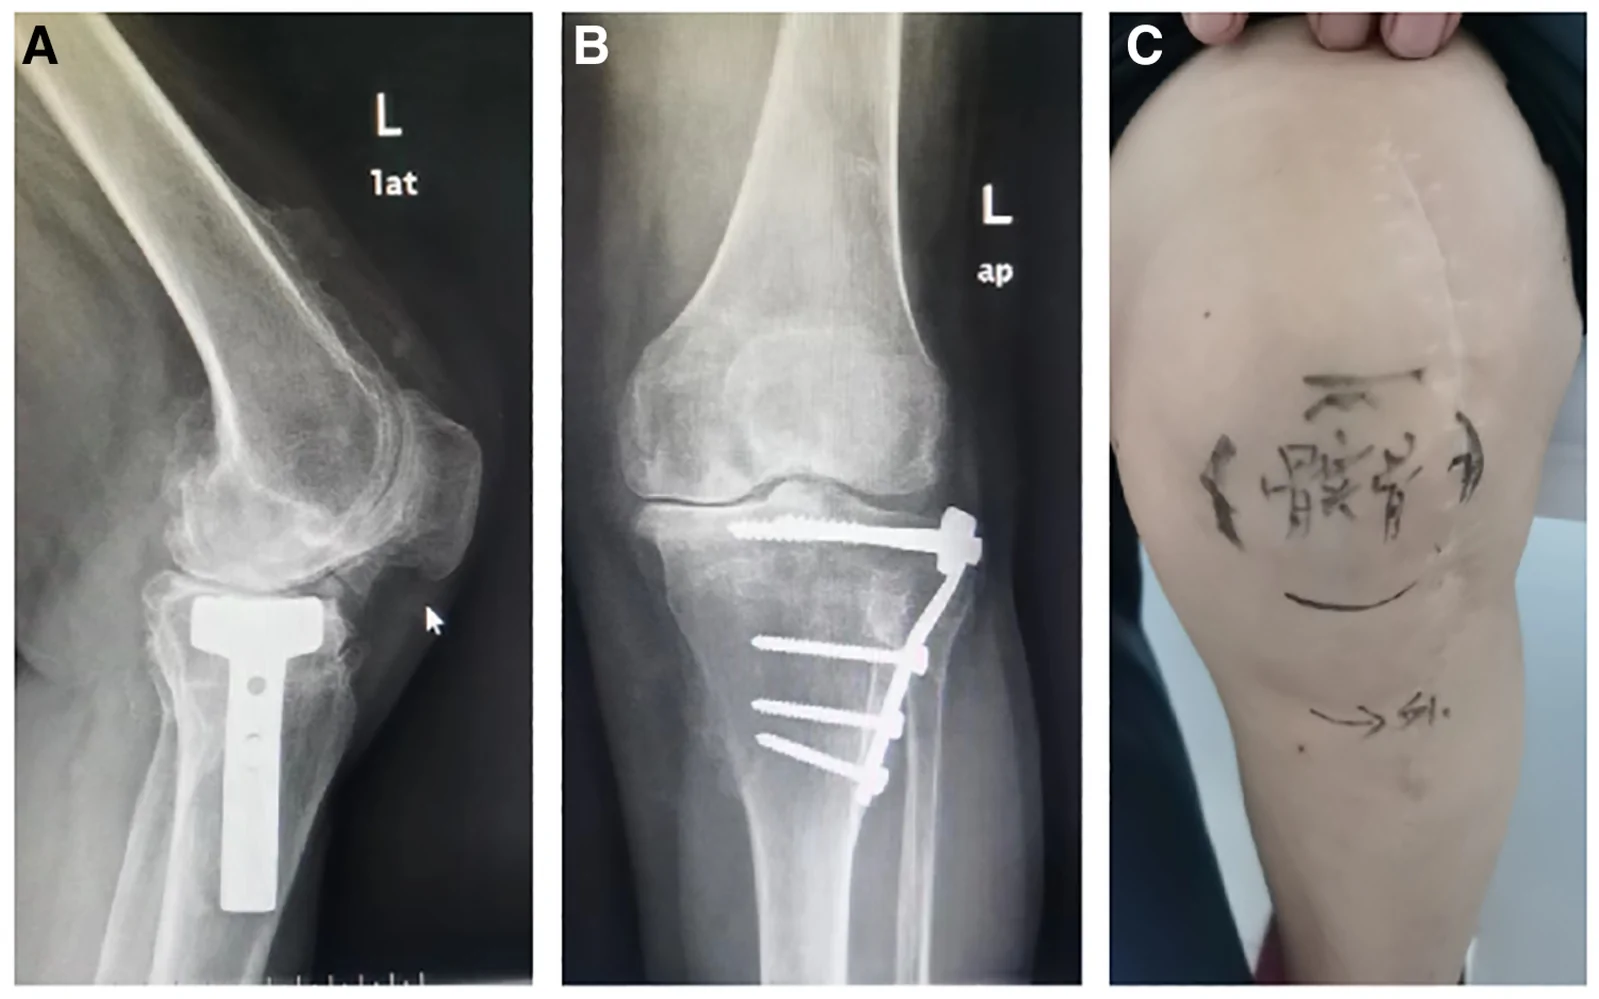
(A) Preoperative anteroposterior view radiograph of the left knee. (B) Preoperative lateral view radiograph of the left knee. (C) Preoperative physical examination revealed a surgical scar on the left lateral knee.
